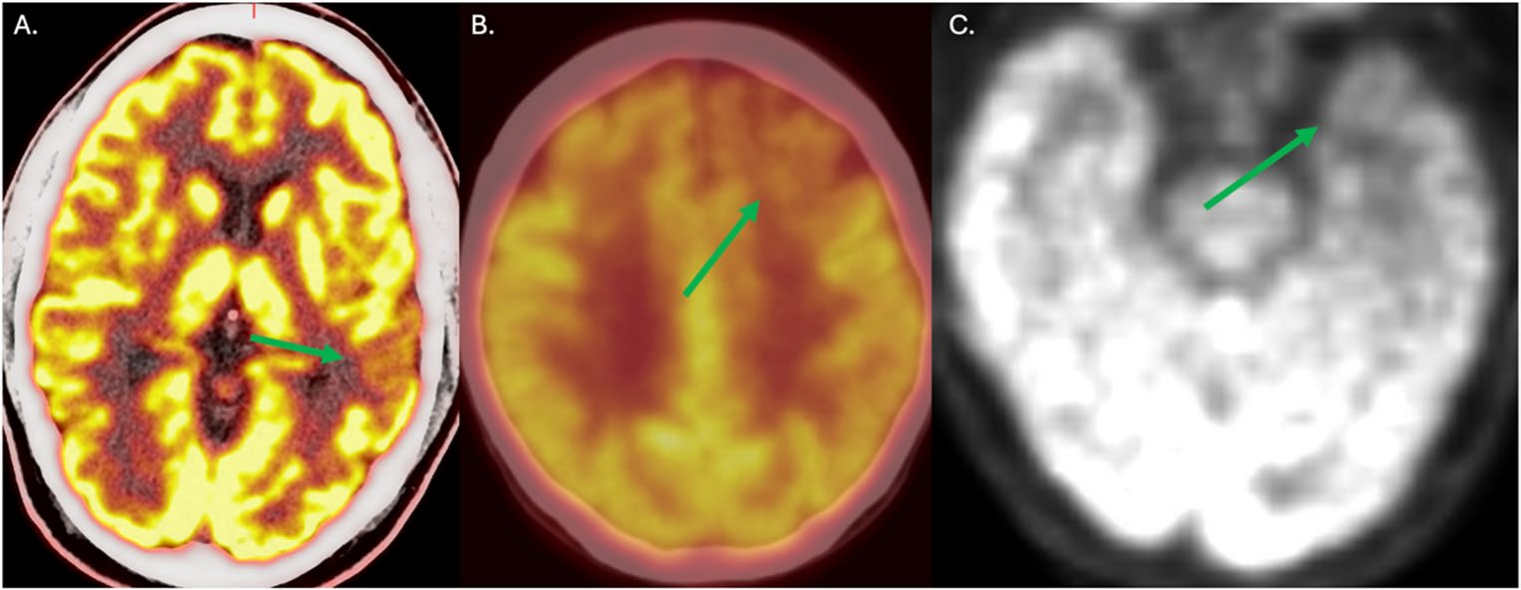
Three patterns of hypometabolism on Flourodeoxy-Glucose Positron Emission Tomography(FDG-PET) in the dominant hemisphere of patients in Northwestern’s longitudinal PPA study with (A) PPA-L, (B) PPA-G, and (C) PPA-S correspond to the anatomy in Fig. 1
